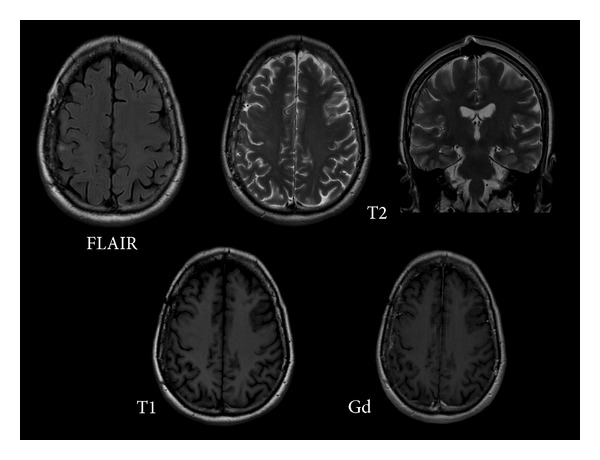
Control brain MRI. Three months after surgery a control brain MRI shows no lesion recurrence.
